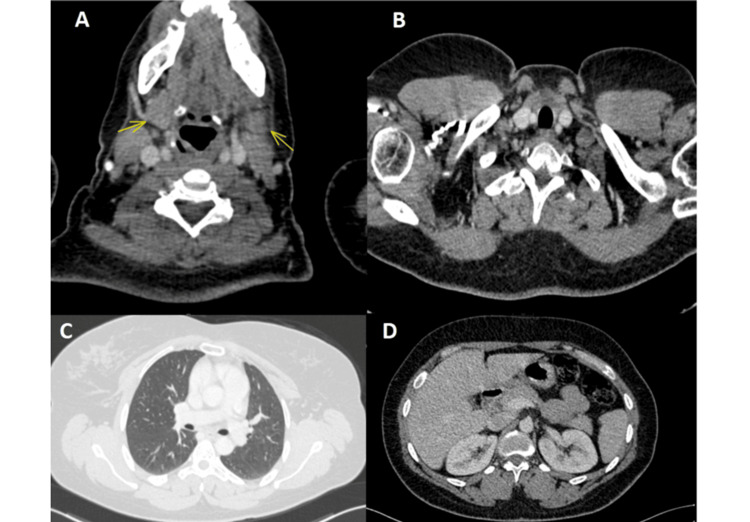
Postoperative cervico-thoraco-abdomino-pelvic CT scan A and B: Axial sections of the cervical region. A shows asymmetry in the size of the submandibular glands related to the surgical procedure. B reveals evidence of thyroidectomy (empty thyroid bed). C: Axial section of the thoracic region, parenchymal window, showing no secondary pulmonary lesions. D: Axial section of the abdominal region, with no secondary lesions detected in this region.
